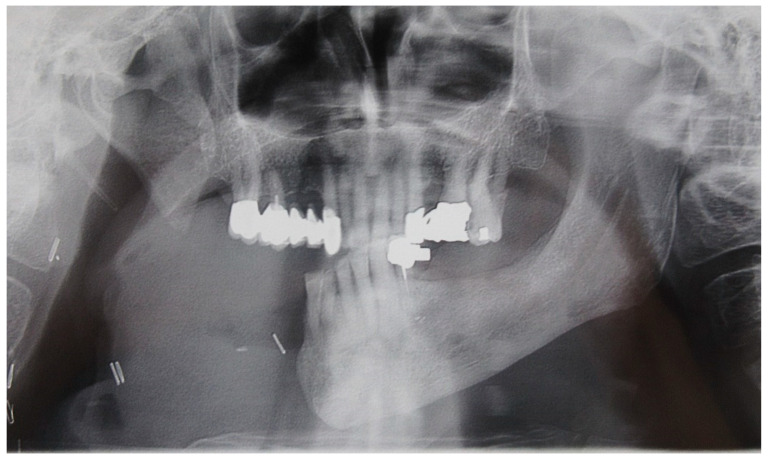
Patient with hemimandibulectomy L2, L3 in orthopanoramic X-ray.
